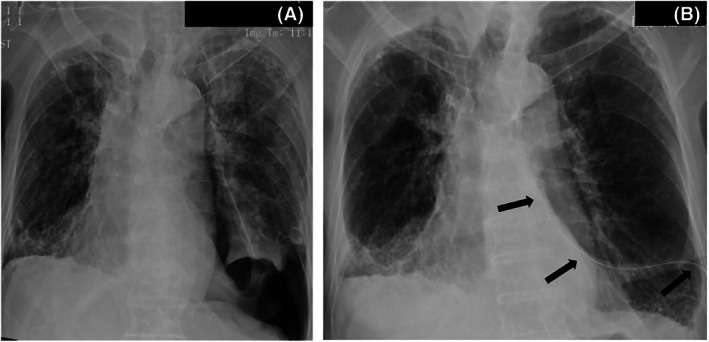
(A) Chest radiograph showing a left pneumothorax. (B) Post–chest tube radiograph demonstrating tube position (arrows) and partial re‐expansion.
